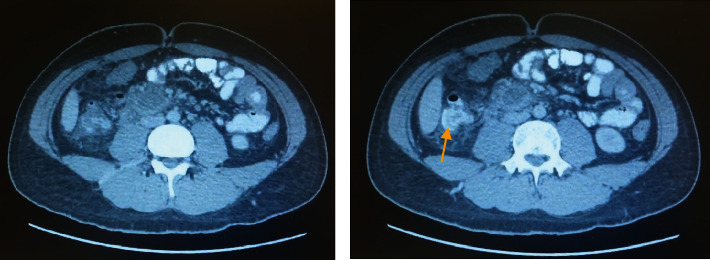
CT fistulography showing fistulous tract between the collection and the third portion of the duodenum (yellow arrow).
